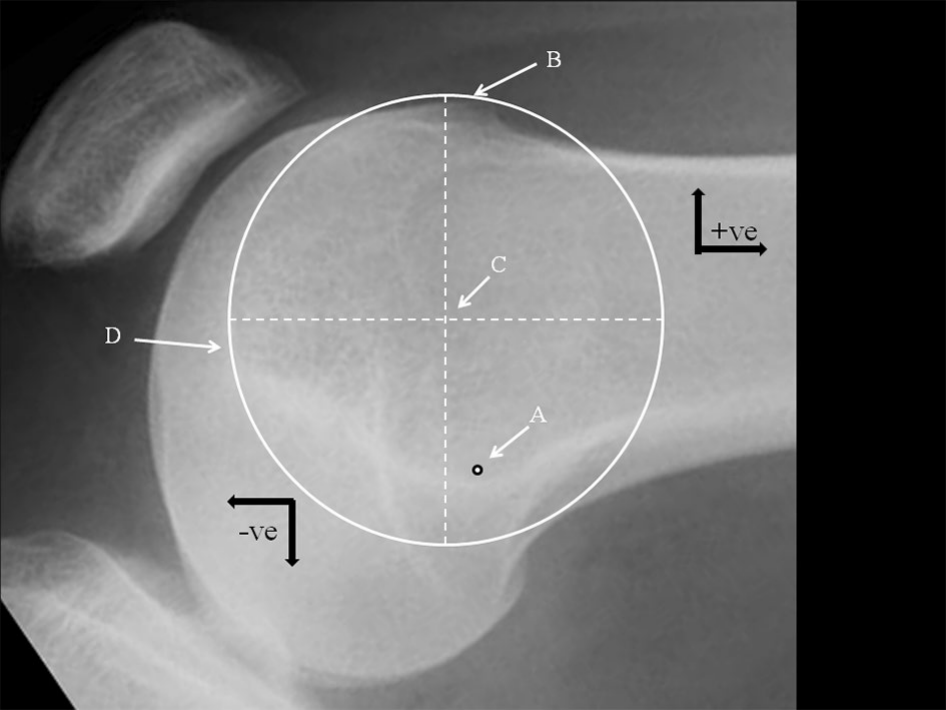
Lateral radiograph of the knee showing: *A* Schöttle’s point. *B* Most anterior point of the groove (also the point where the boss height is measured). *C* Isometric point (centre of the *circle* that includes the groove). *D* Most distal point of the groove. Black arrows indicate convention chosen for defining relative position of a point
